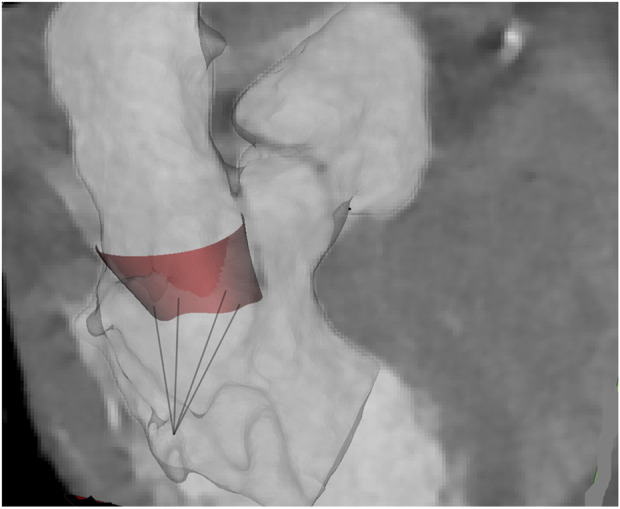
Representative shape of MV and chordae at atrial systole as superimposed onto the original angio-CT images.
